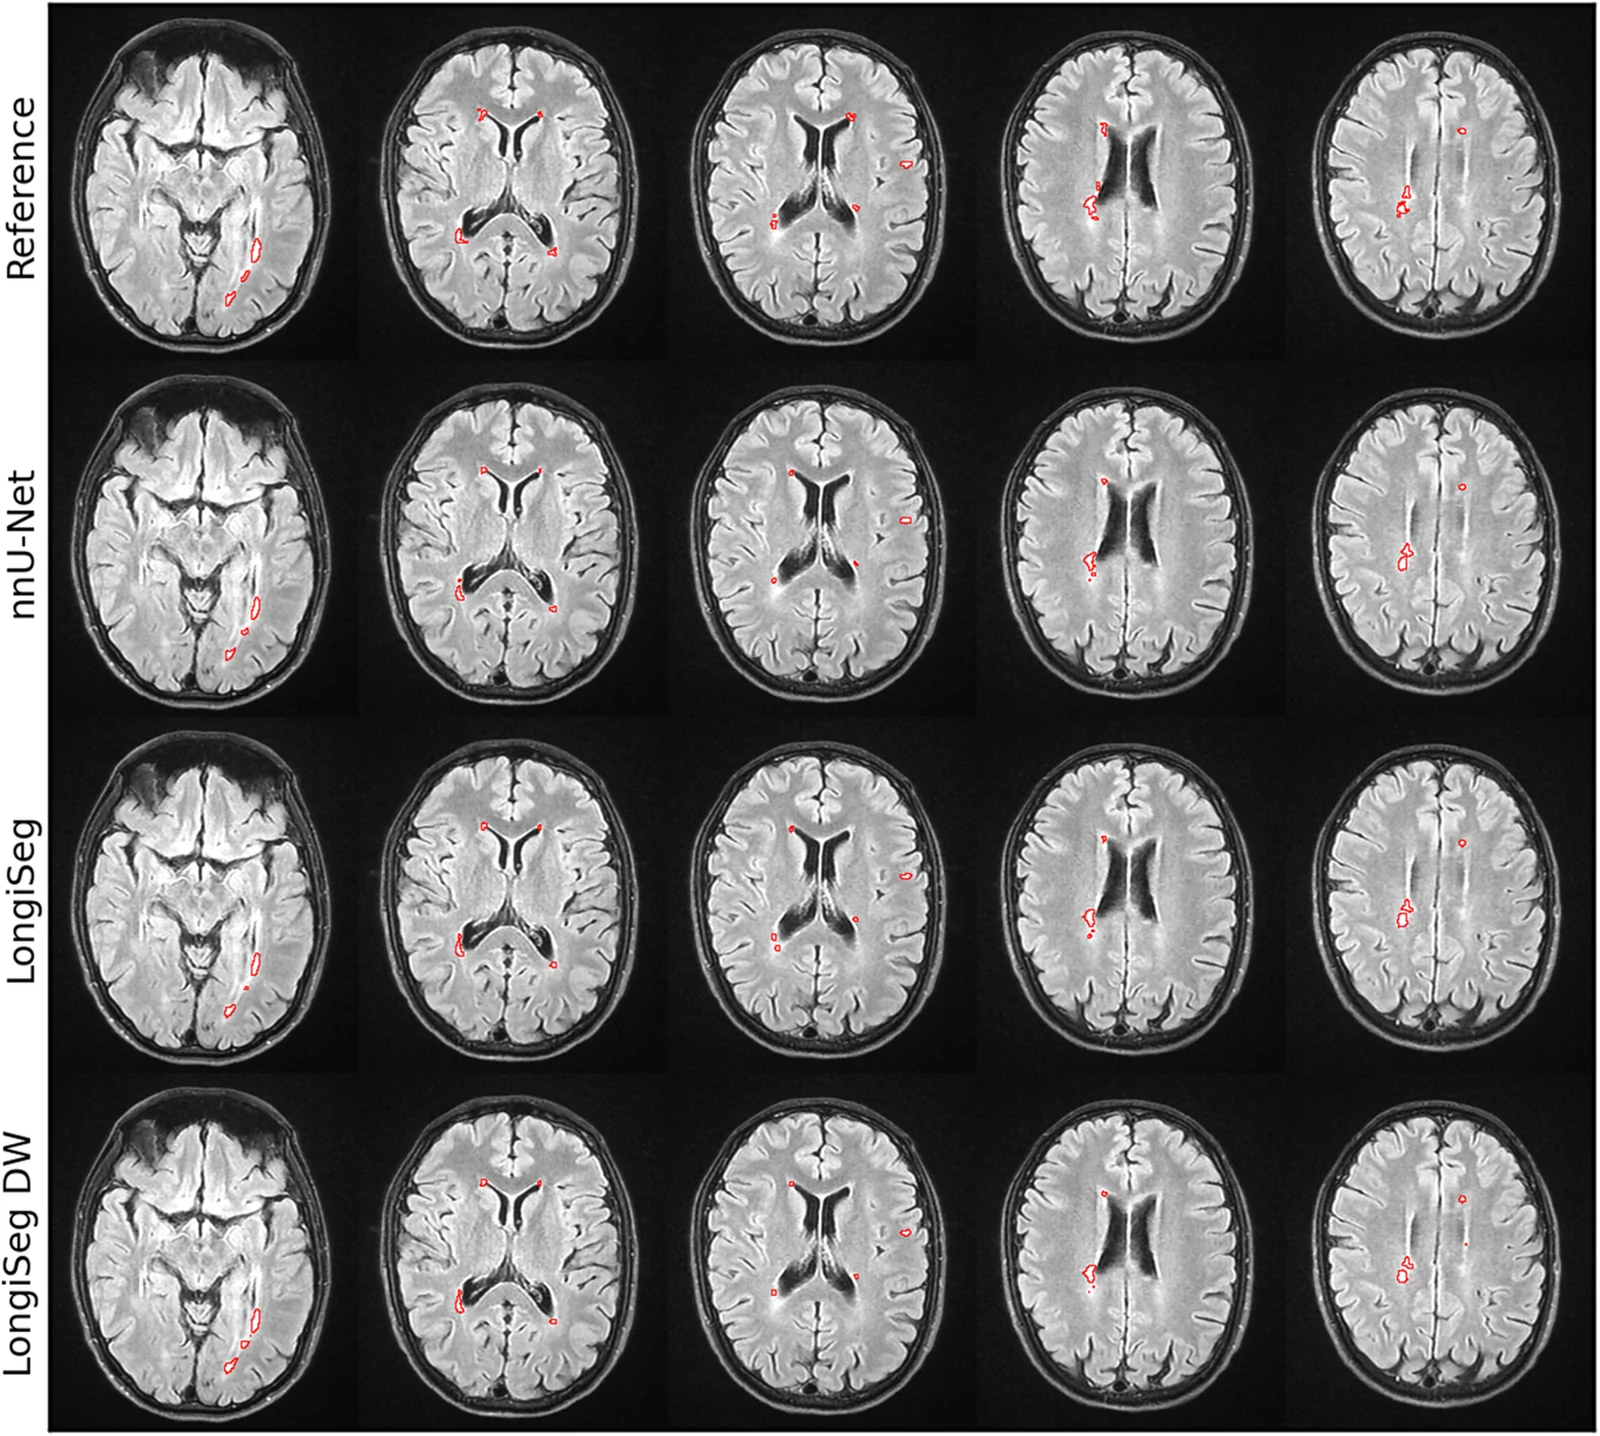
Example slices with delineations made by the single-input nnU-Net, LongiSeg and LongiSeg with difference weighting (DW), on a patient from the clinical test dataset. All three models are trained with T2w FLAIR input only. Though nnU-Net performed significantly worse on the average segmentation performance, there are minimal visible differences in the delineations on the example patient, indicating modest performance gains when changing to LongiSeg.
